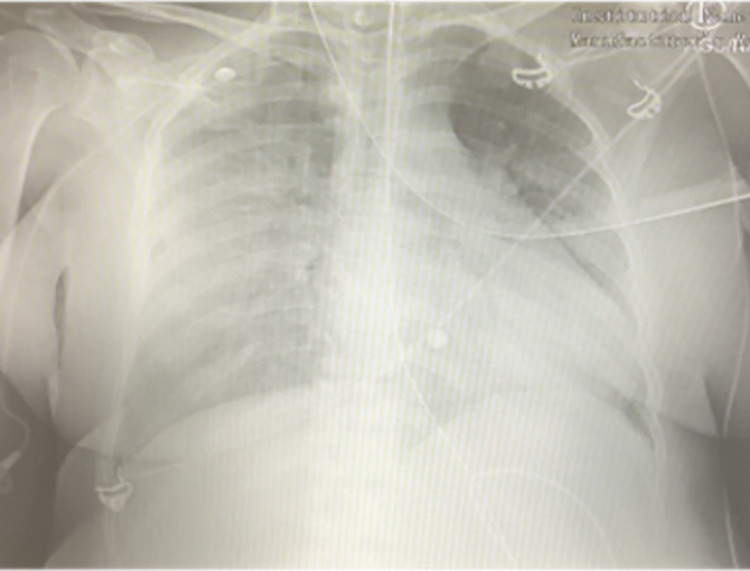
Chest X-ray showing hazy infiltrates with airspace disease throughout the right lung as well as in the left middle and lower lung consistent with viral pneumonia.
